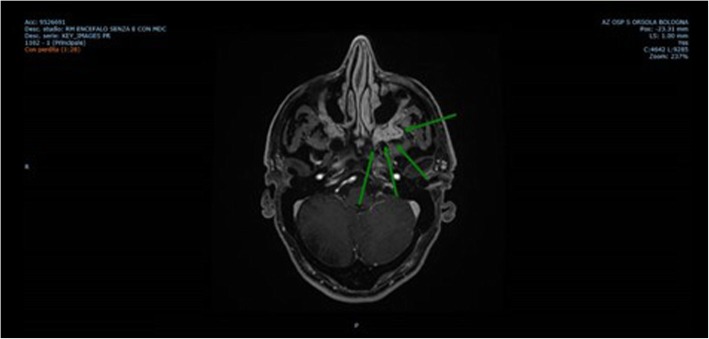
Pathological lesion biopsied (indicated by arrows) on Assial MRI 3DT1 fat saturated post-contrast sequence
